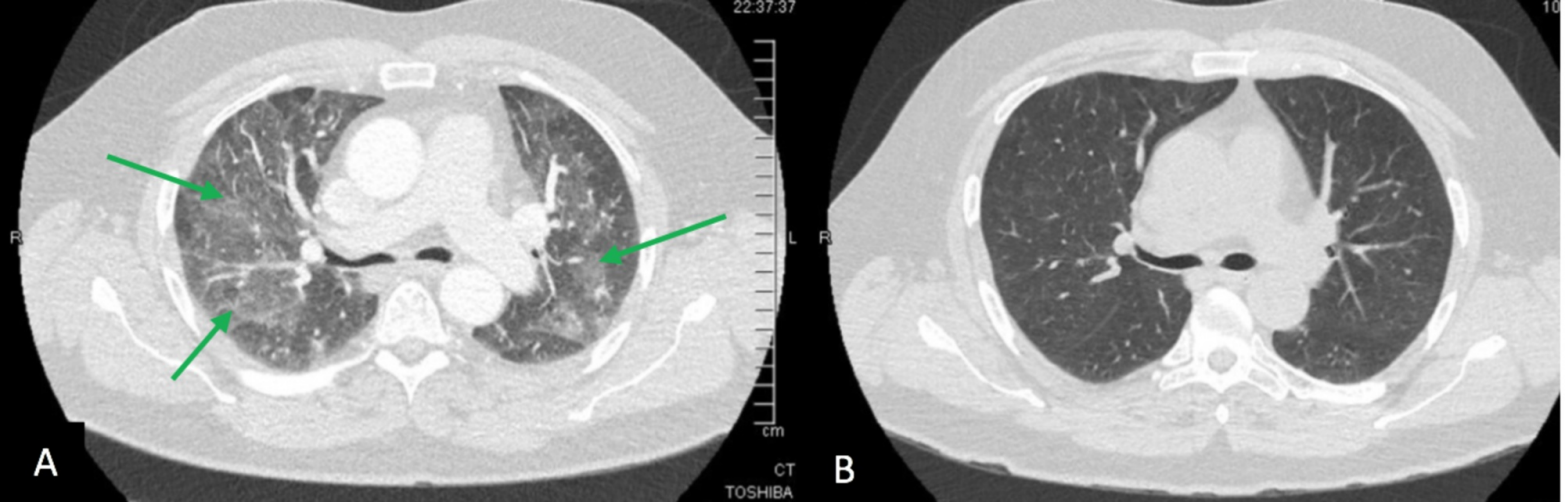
CT chest axial images showing A) severe TM in distal airway involving both mainstem bronchi and ground glass opacities (green arrows) bilaterally vs. B) improvement in TM with now patent normal caliber mainstem bronchi bilaterally and nearly resolved GGO. TM, tracheomalacia; GGO, ground glass opacities
